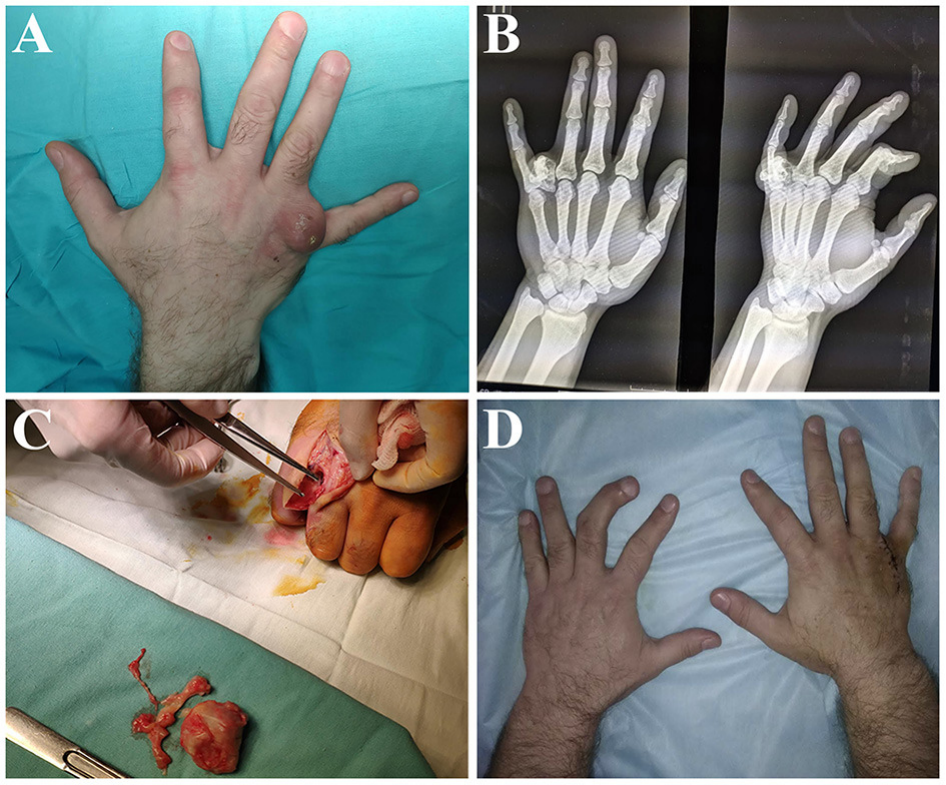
Diagnostics and surgical treatment. (A) Macroscopic observation of the hand on admission: a round infiltrated formation with a fistulous opening was located at the base of the ring finger. (B) X-ray of the hand on admission: an enchondroma with an heterogenous structure was visualized at the base of the proximal phalanx. (C) Intraoperative macroscopic revision of necrotic bone and soft tissues. (D) Macroscopic observation of the hand upon discharge from the hospital: wound healed by primary intention.
